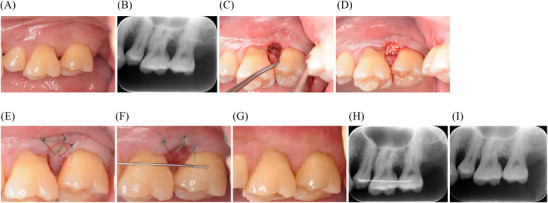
Clinical and radiographic presentation of periodontal regenerative therapy using M‐MIST in the maxillary molar region. (A) Preoperative clinical view of the interdental area between teeth #14 and #15. (B) Preoperative radiograph showing vertical bone defect. (C) Combined bone defect (1‐wall coronal and 2‐wall apical). (D) Placement of carbonate apatite (Cytrans granules). (E) Immediately postoperative buccal view showing primary closure. (F) Buccal view showing primary healing at 1‐week postoperative. (G) Clinical presentation at 1.5‐year follow‐up. (H) Immediately postoperative radiograph. (I) 1.5‐year follow‐up radiograph showing bone fill. M‐MIST, modified minimally invasive surgical technique.
